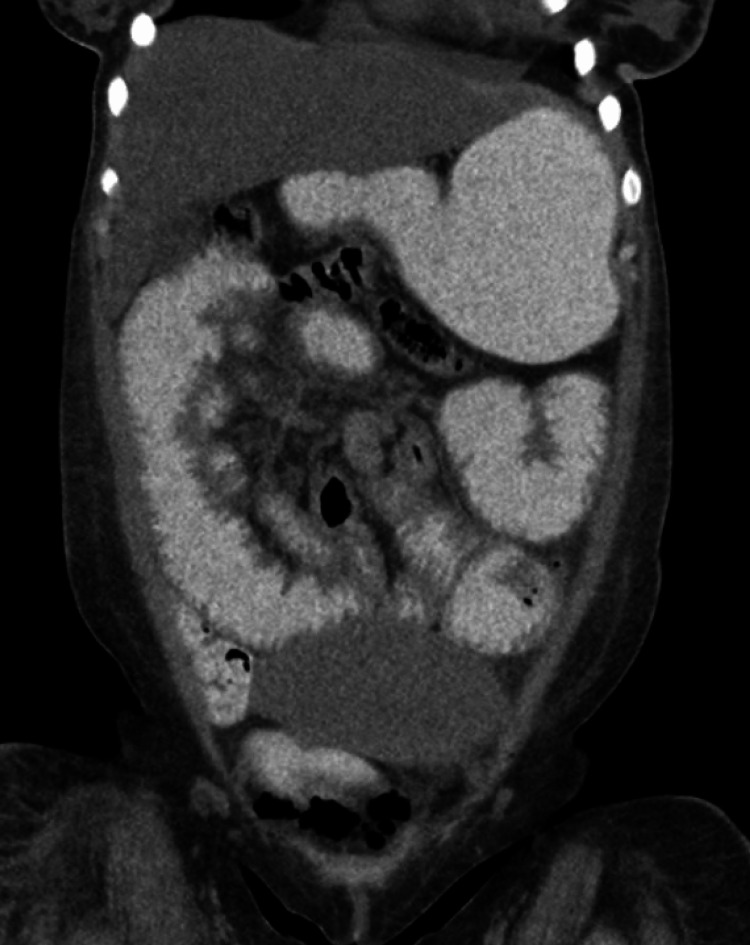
CT Abdomen with Oral Contrast Computed tomography (CT) abdomen with oral contrast showing a transition point and proximal dilated bowel with distal decompressed bowel, concerning intestinal obstruction.
